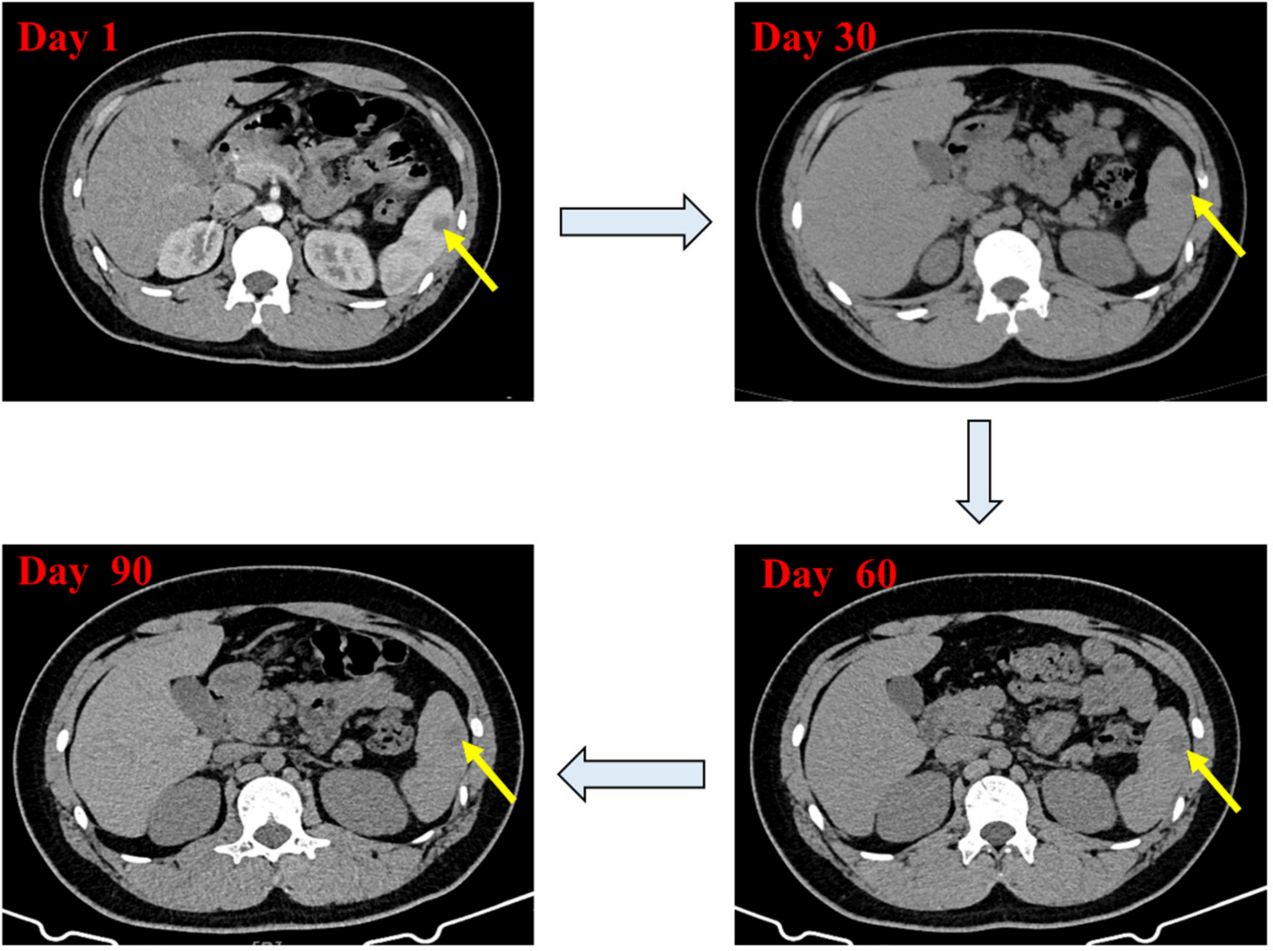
Contrast-enhanced abdominal CT scan showing changes in the low-density lesion in the spleen during treatment.
